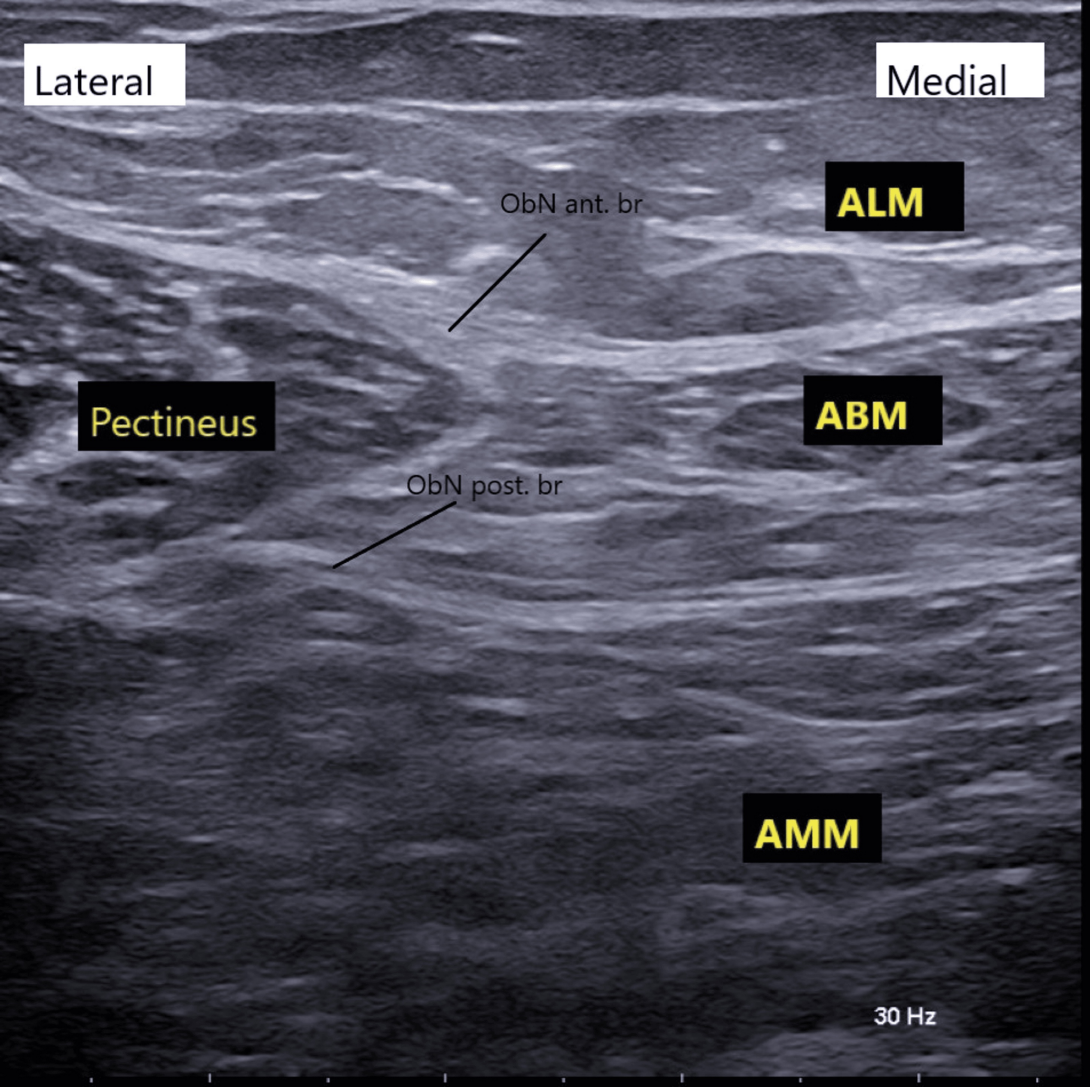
Ultrasonographic anatomy of the obturator nerve ALM, adductor longus muscle; ABM, adductor brevis muscle; AMM, adductor magnus muscle; Ob.N Ant.Br., obturator nerve anterior branch; Ob.N post.Br, obturator nerve posterior branch; Pectineus: pectineus muscle.
